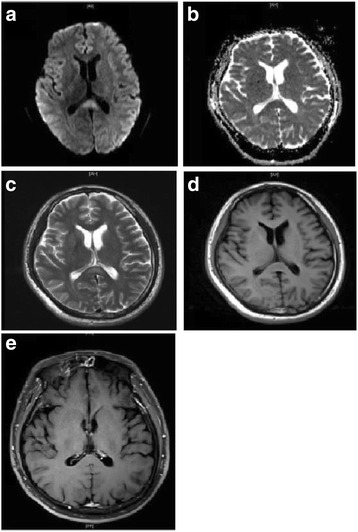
Initial cranial MRI of the patient. The lesion in the midline of SCC was hyperintensity on DWI (a) and T2WI (c), decreased ADC value (b), isointense signals on T1WI (d), and no contrast enhancement (e)
